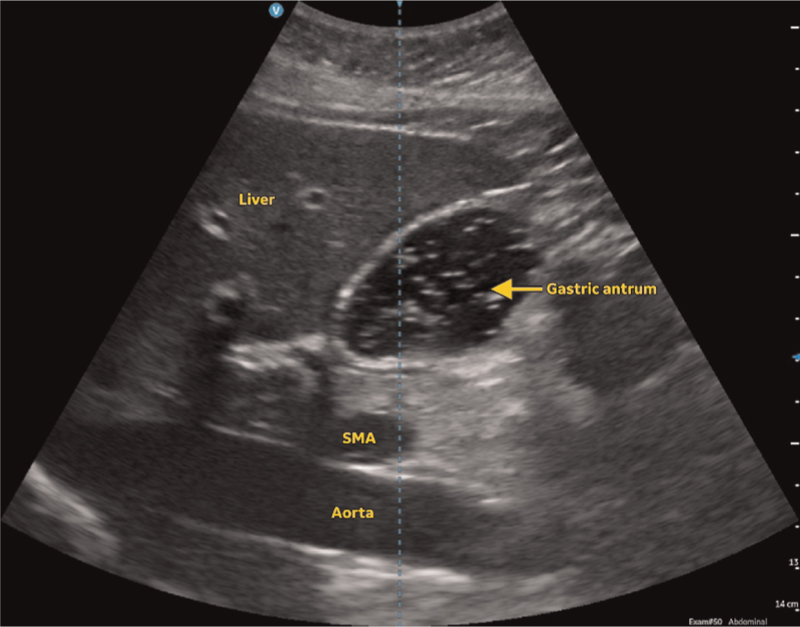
Ultrasound image of the gastric antrum following the ingestion of fluids in a patient in the first trimester of pregnancy.
